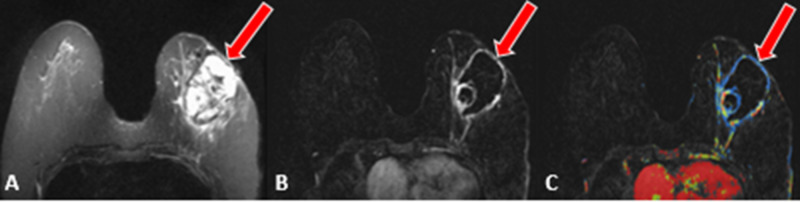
Case 5 breast MRI findings A: Axial T2-weighted image shows a heterogeneous mass with areas of T2 hyperintensity. B, C: Axial T1-weighted fat-suppressed subtracted without (B) and with (C) color show a heterogeneously enhancing centrally necrotic mass in the left breast (red arrows).
